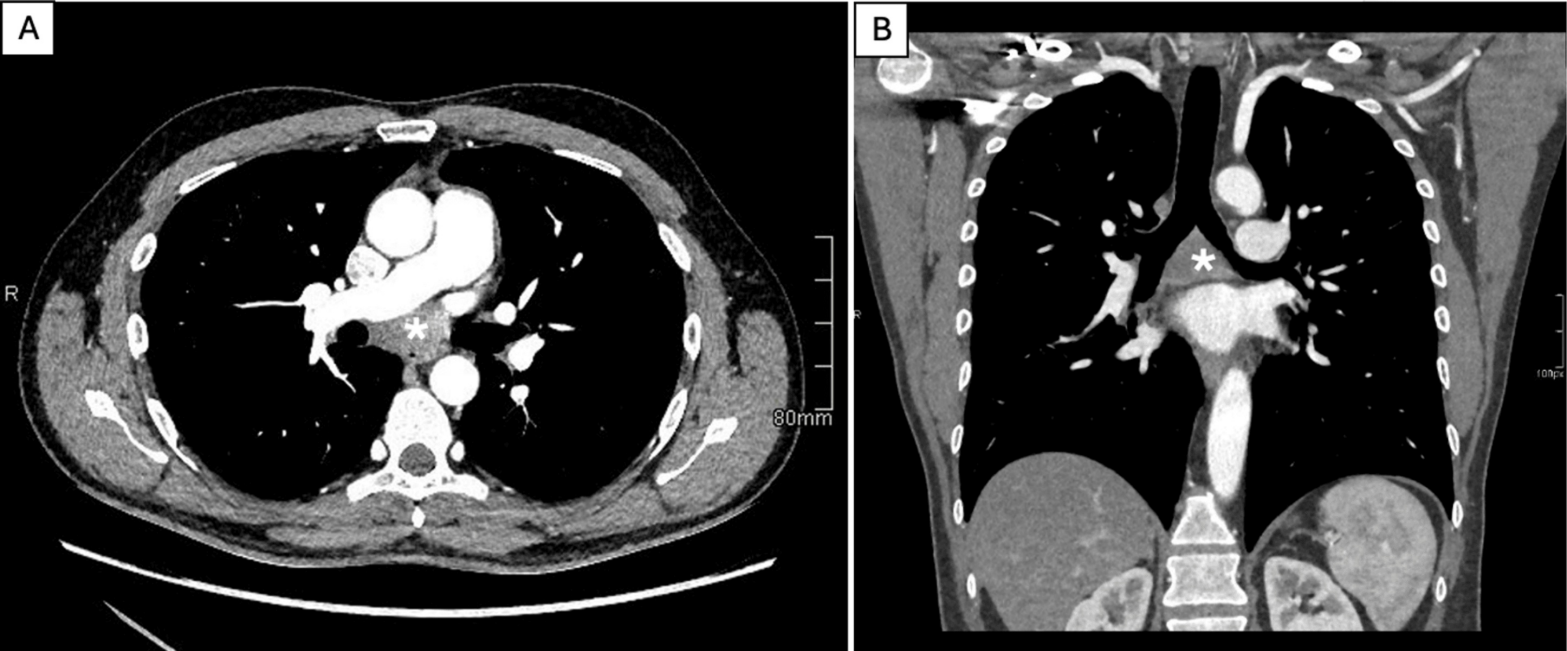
Computed tomography (CT) at the six-month follow-up Six months following excision, a persistent residual lesion of approximately 3.5 cm width (white asterisk) is noted in the subcarinal space with internal fluid contents of variable density (30-60 Hounsfield units), possibly representing a remnant of the primary bronchogenic cyst.
